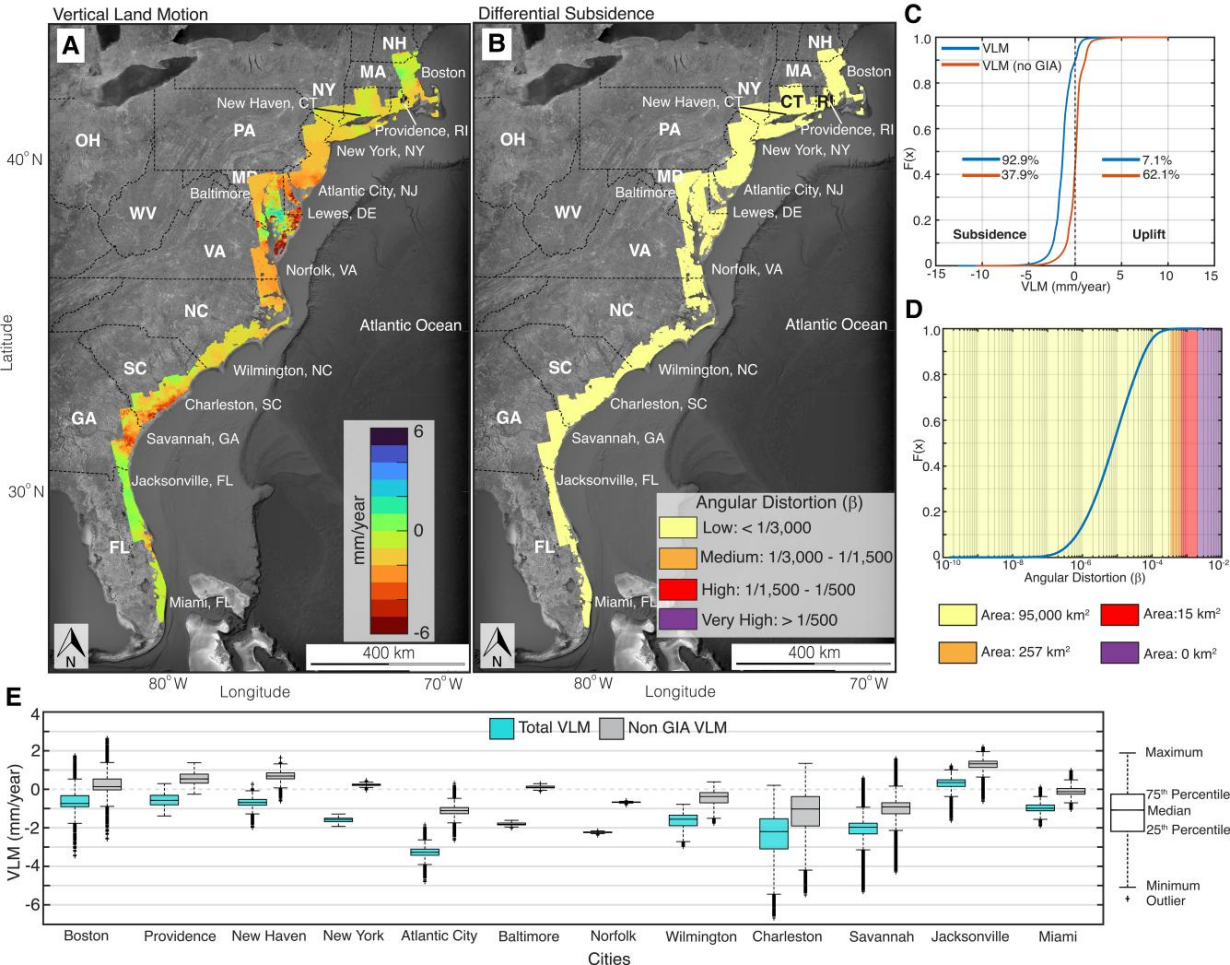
Vertical land motion (VLM) along the US east coast. A) Updated VLM rate for the US east coast from this study. This map includes ∼25 million pixels at ∼50 m resolution. B) Spatial distribution of angular distortion (*β*) map. Background Images in (A) and (B) are from Google, Earthstar. C) Empirical cumulative distribution function for VLM and VLM without GIA. The GIA data from the ICE-6G-D model (42). D) Empirical cumulative distribution function for *β*. The area for each *β* category is estimated using a regular grid of 50 m. E) Distribution of land subsidence for 12 metropolitan cities on the US east coast: Boston (MA), Providence (RI), New Haven (CT), New York (NY), Atlantic City (NJ), Baltimore (MD), Norfolk (VA), Wilmington (NC), Charleston (SC), Savannah (GA), Jacksonville (FL), and Miami (FL). The locations of the cities are highlighted in (A).
